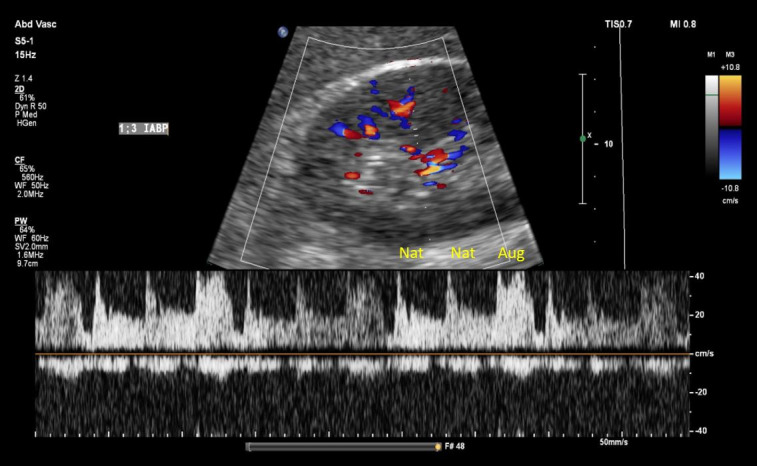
Intrarenal Doppler at intra-aortic balloon pump (IABP) frequency of 3:1. Nat, native arterial beat; Aug, balloon augmented flow.
